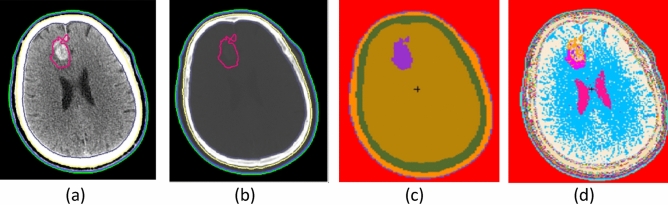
Brain tumor case: (a) CT image in the brain window. (b) CT image in the bone window. (c) Conversion model I with specific material from the ICRU-46 report in ROIs. (d) Conversion model II with multiple materials that were automatically converted to the elemental compositions and densities by the HU values.
